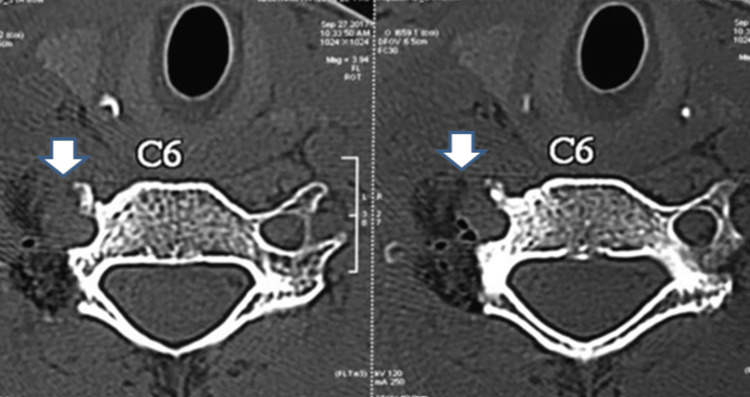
Intraoperative CT shows complete excision of the tumor, indicated by arrows CT: computed tomography; C6: sixth cervical vertebra
